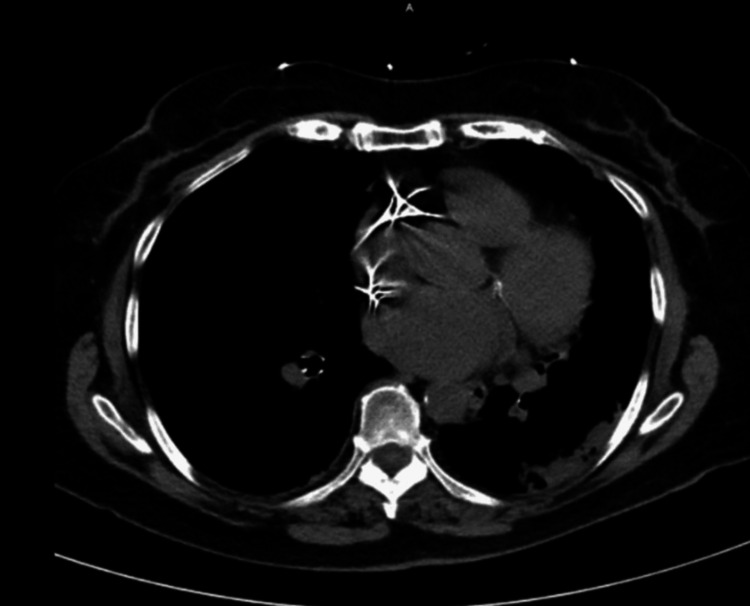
CT chest confirms left lower lobe consolidation, consistent with pneumonia.
